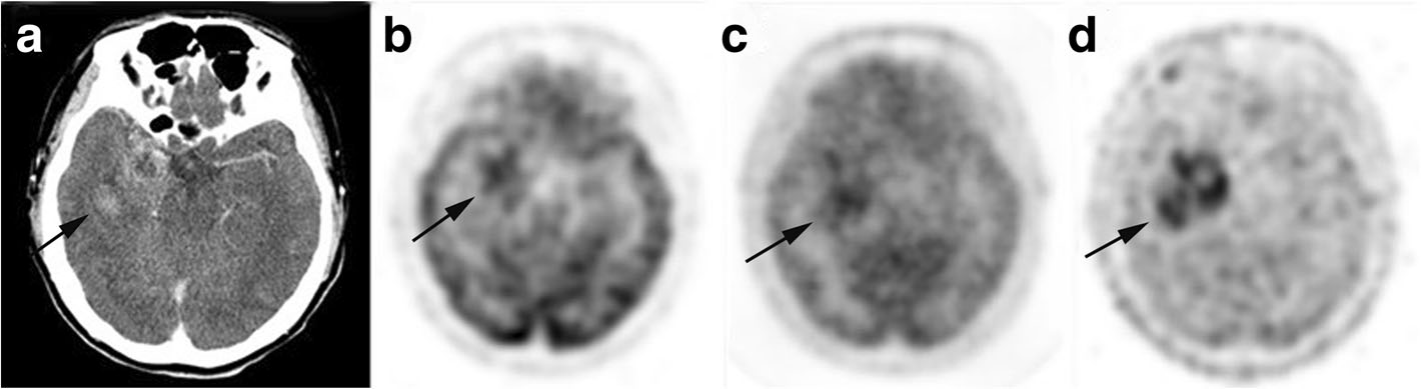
A patient aged 40–45 years with glioblastoma. Contrast enhanced CT (a) shows a moderately enhanced lesion in the right temporal lobe (arrow). ^18^F-FDG (b), ^13^N-NH_3_ (c) and ^11^C-MET (d) were all positive for the lesion, and the tracer accumulation of ^11^C-MET was higher than that of ^13^N-NH_3_ and ^18^F-FDG
